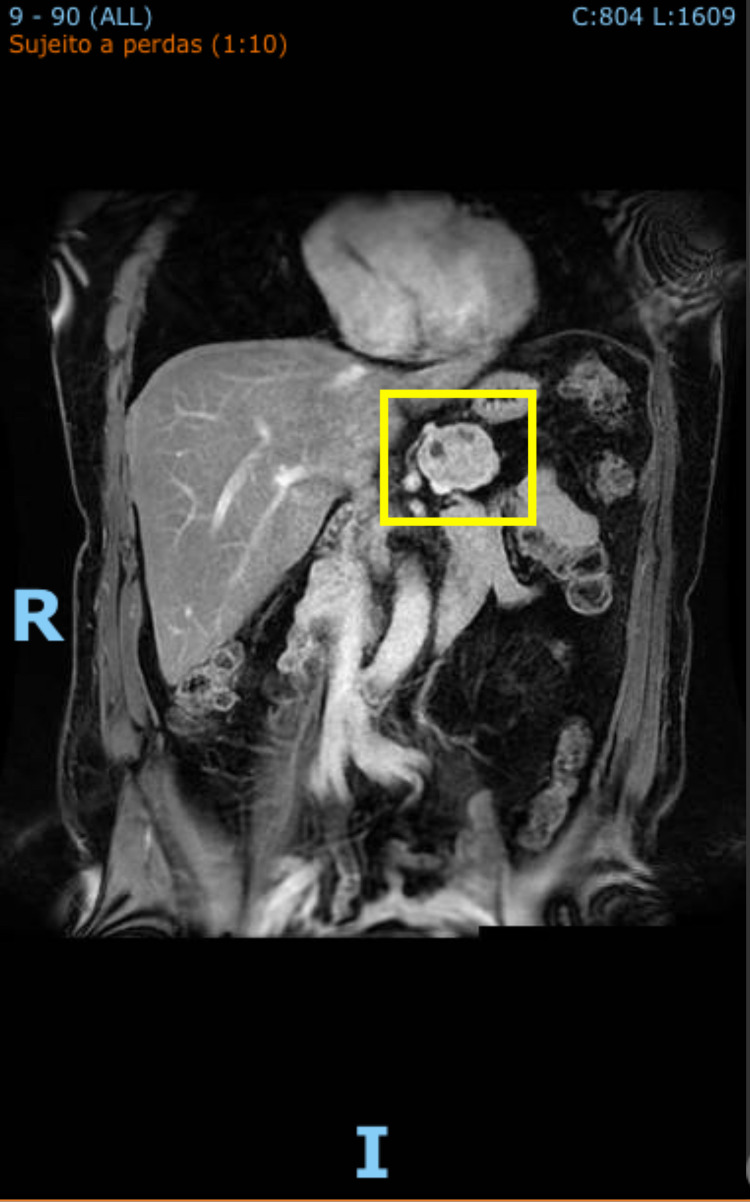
MRI abdomen and pelvis with contrast; lesion marked. Lesion noted to the body of the pancreas.
